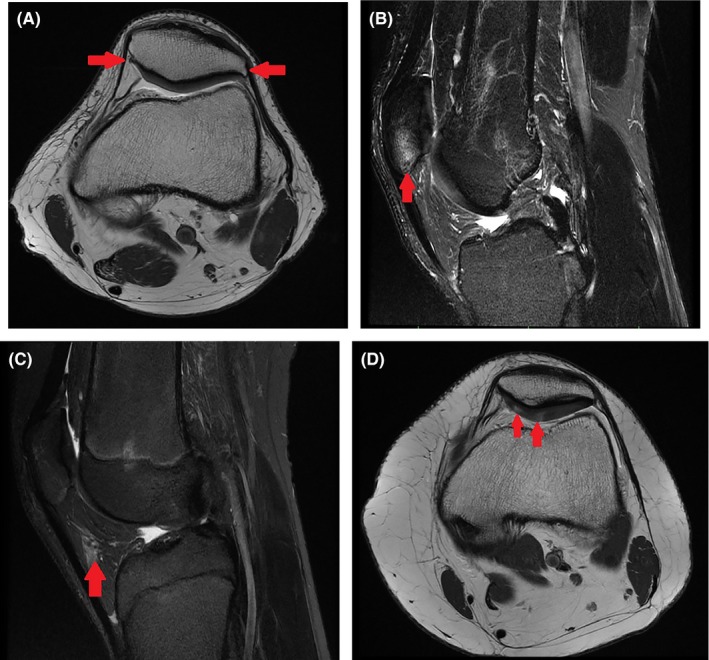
Structural abnormalities. A, Osteophytes on patella (axial proton density weighted MRI); B, Bone marrow lesions in patella (sagittal T2‐weighted fat‐saturated MRI); C, Hoffa‐synovitis (sagittal T2‐weighted fat‐saturated MRI); D, Minor cartilage defects in patellar cartilage (axial proton density weighted MRI). Arrows highlight affected areas
